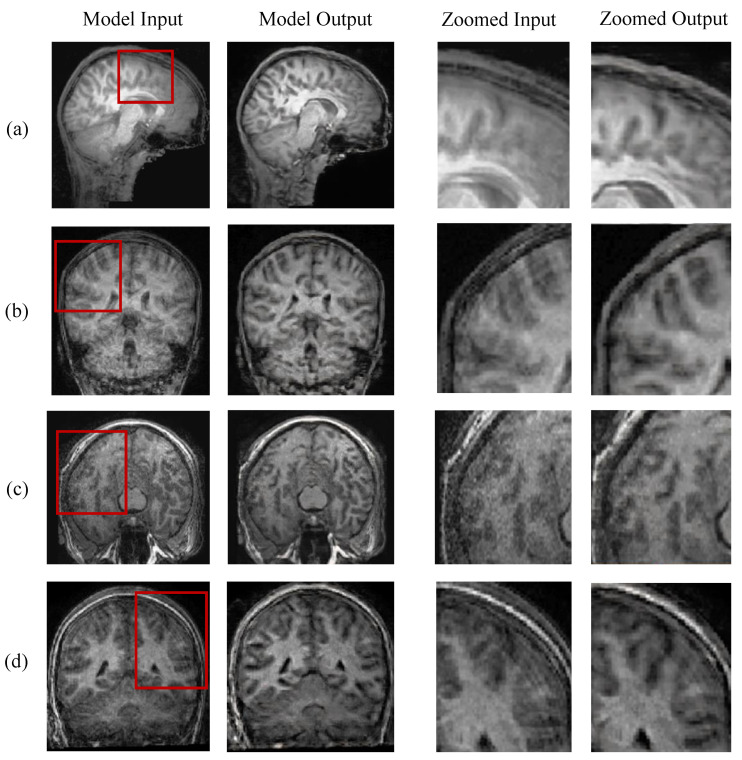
Visual Assessment of MC-GAN on Real-world Motion-affected Images. Images under the “Model Input” columns are original MR images; model-corrected output is displayed to the right. The two columns on the right show the zoomed-in regions indicated by the red boxes. Samples are selected from the sagittal (a), coronal (b,d), and axial (c) directions.
